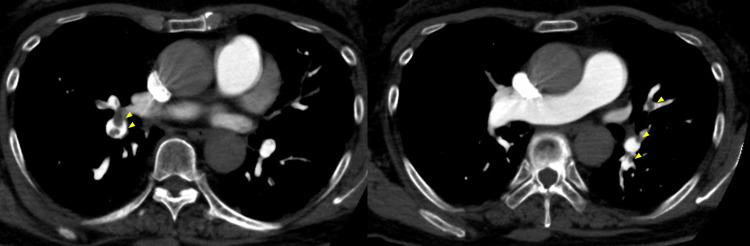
Computed tomography angiography findings in Case 2 Chest computed tomography angiography demonstrated multiple sites of blood flow interruption in bilateral pulmonary arteries (arrowheads).
